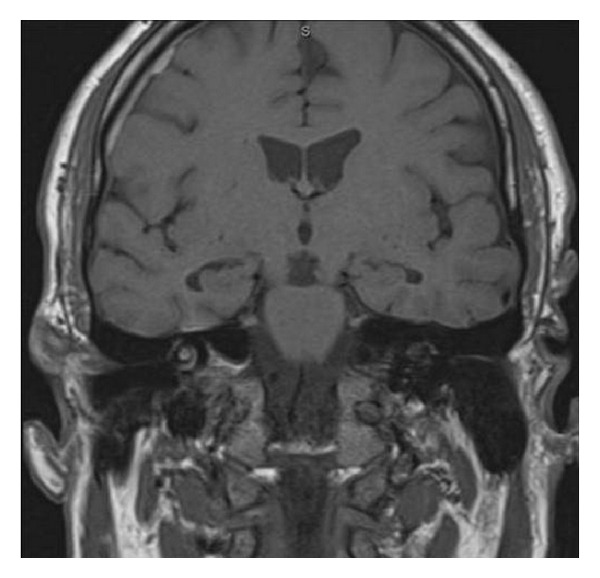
Brain MRI showing hypersignal in the inner ear compatible with hemorrhagic labyrinthitis.
